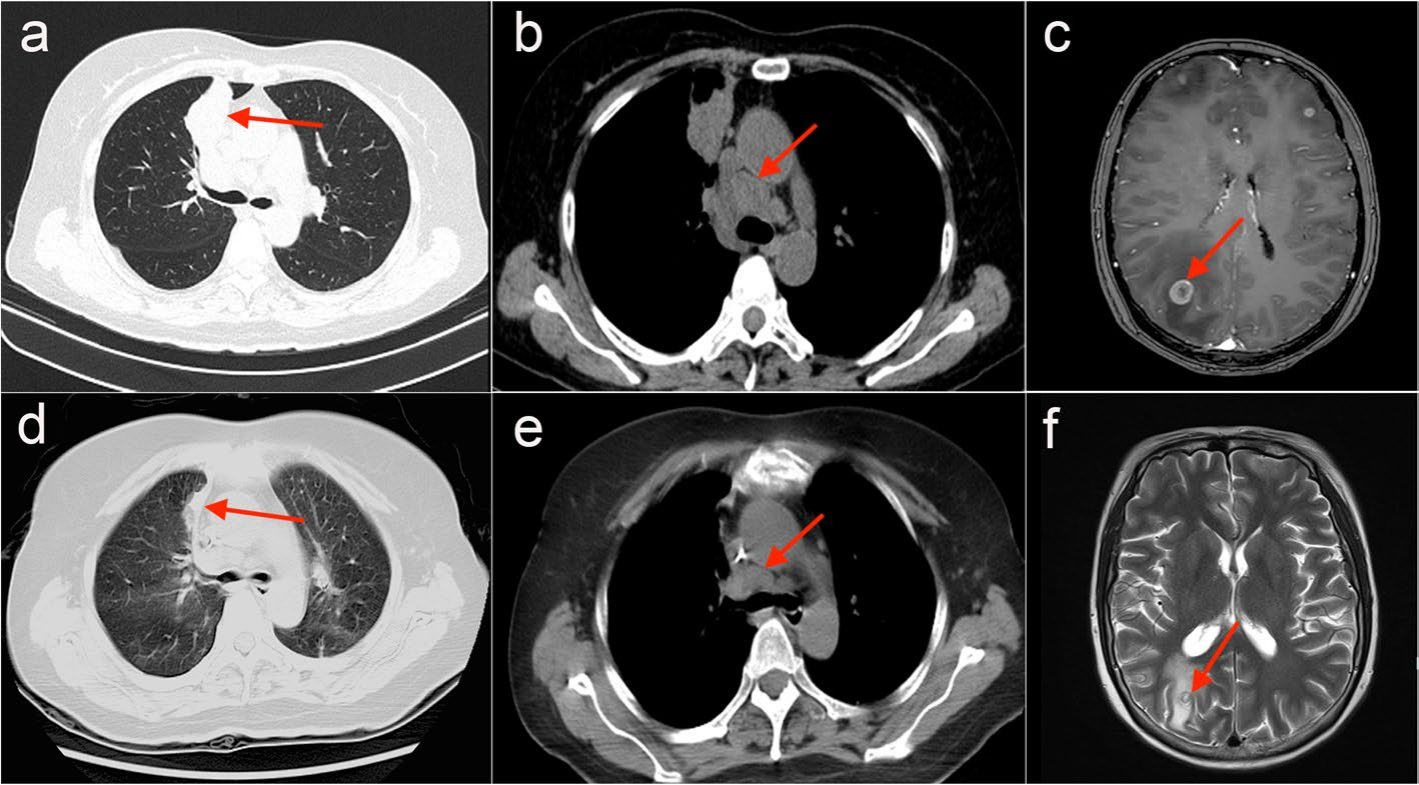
The image changes before and after treatment. The CT scan before treatment showed the mass in the right upper lobe sized 4.6 × 3.2 cm (a), mediastinal 4R enlarged lymph nodes (b). Contrast enhanced MRI of brain before treatment revealed multiple intracranial space-occupying lesions (c). The CT examination after treatment with pralsetinib displayed the mass sized 2.8 × 1.2 cm in the right upper lobe (d), marked reduction in the size of lymph node in mediastinum 4R (e). Brain MRI after treatment demonstrated the shrink of the right parieto-occipital region (f). Red arrows indicate the tumor or lymph node lesions
